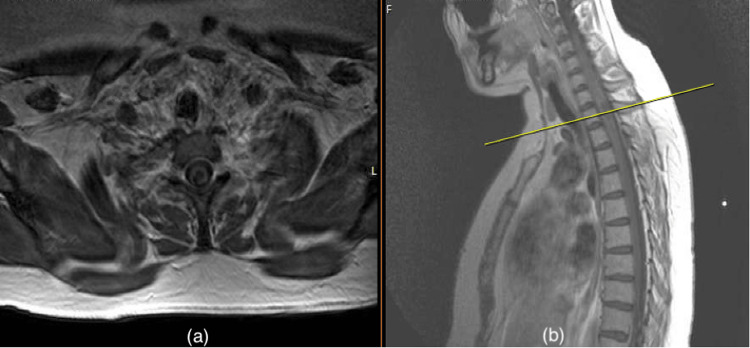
T2 sequenced MRI images T2 sequenced of MRI (a) axial section and (b) sagittal section of the whole spine showing an intrinsic intramedullary vascular lesion from level C6 down to T2 (as marked by the yellow line) MRI: Magnetic resonance imaging
